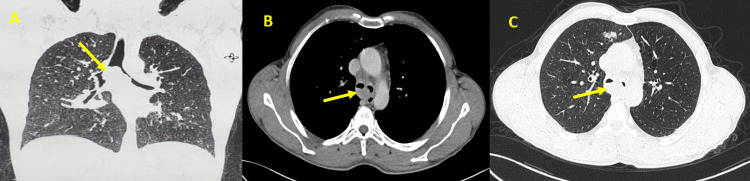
CT scan images CT scan reveals a luminal narrowing due to intratracheal tissue mass (arrows). Figure A shows the coronal view with a parenchymal window, Figure B displays the axial view with a mediastinal window, and Figure C presents the axial view with a parenchymal window.
